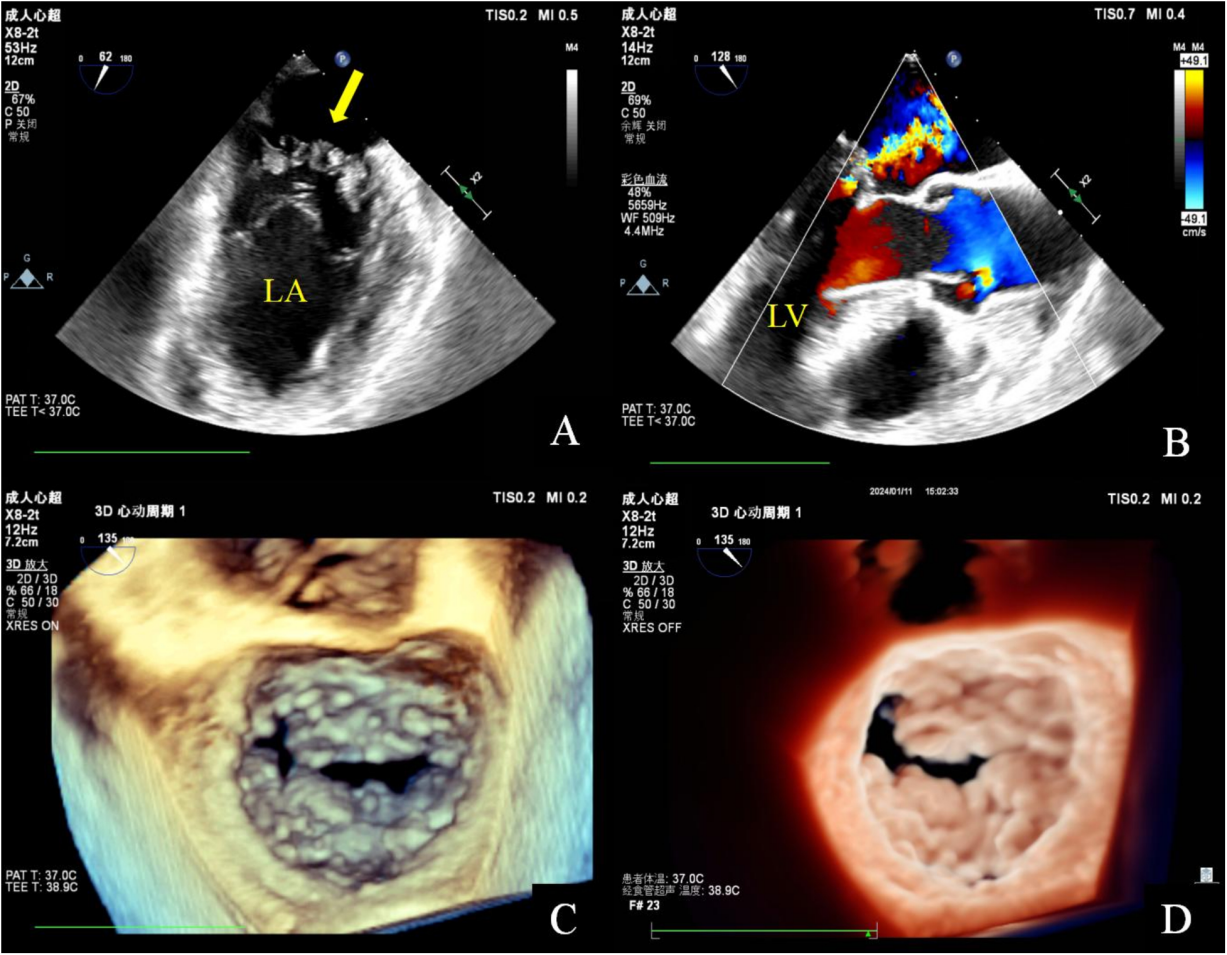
Transesophageal echocardiography. Mid-esophageal mitral commissural view: multiple irregularly shaped masses with a broad base attached to the anterior and posterior mitral leaflets. These masses are characterized by non-smooth surfaces, with an uneven internal echo (A). Mid-esophageal long axis view: color doppler flow imaging indicated that the lesion has a soft and deformable texture, leading to moderate mitral regurgitation (B). Three-dimensional transesophageal echocardiography: a diffuse distribution of granular additional echoes on the left atrial surface in two-thirds of the area of the anterior and posterior leaflets of the mitral valve, as well as the commissural cusps. The lesions merge with each other and are characterized by a wider base and a rough surface (C,D).
